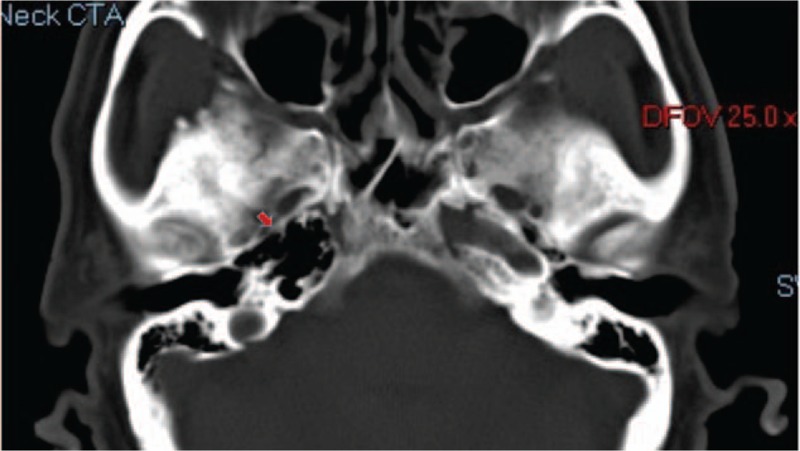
CT image of the skull base showing the complete absence of the right carotid canal with extension of the pneumatization of the right petrous bone (arrowhead), consistent with congenital ICA agenesis.
